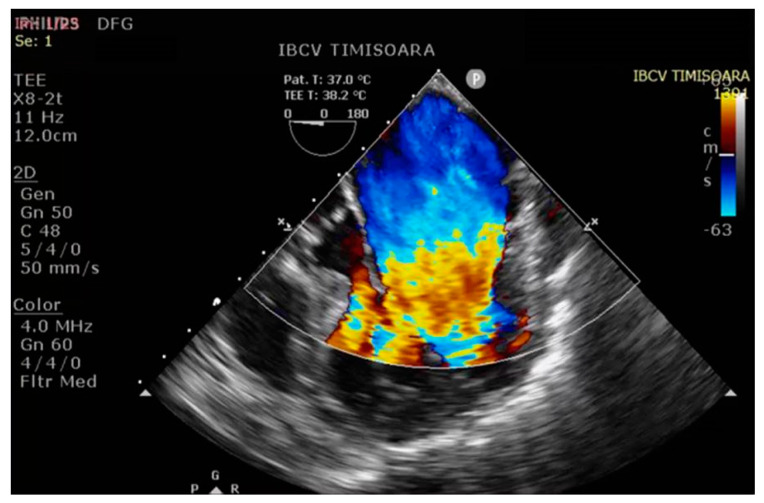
Color Doppler aspect of the repaired mitral valve.
